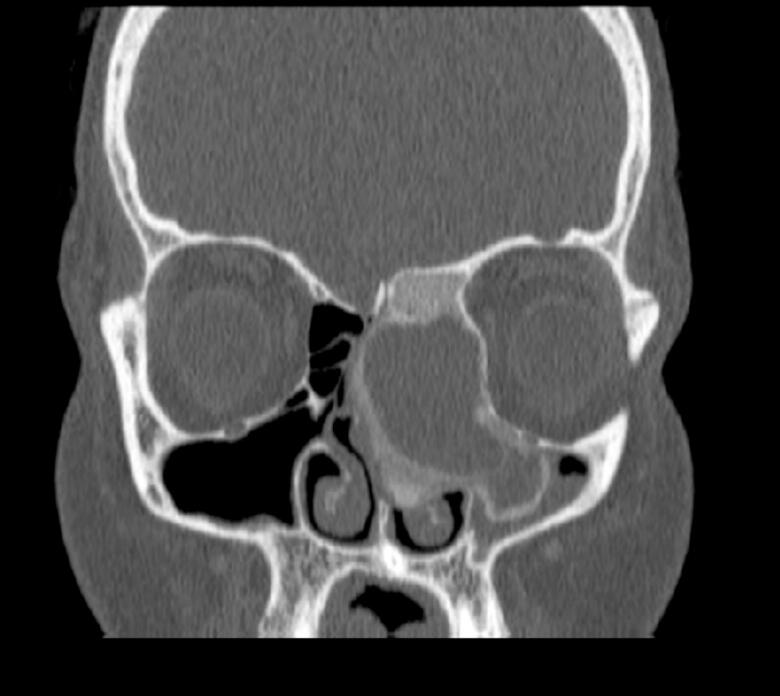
Coronal view Computed Tomography (CT) of paranasal sinuses showing left sided nasal cavity lesion with peripheral calcification extending to left maxillary sinus.
